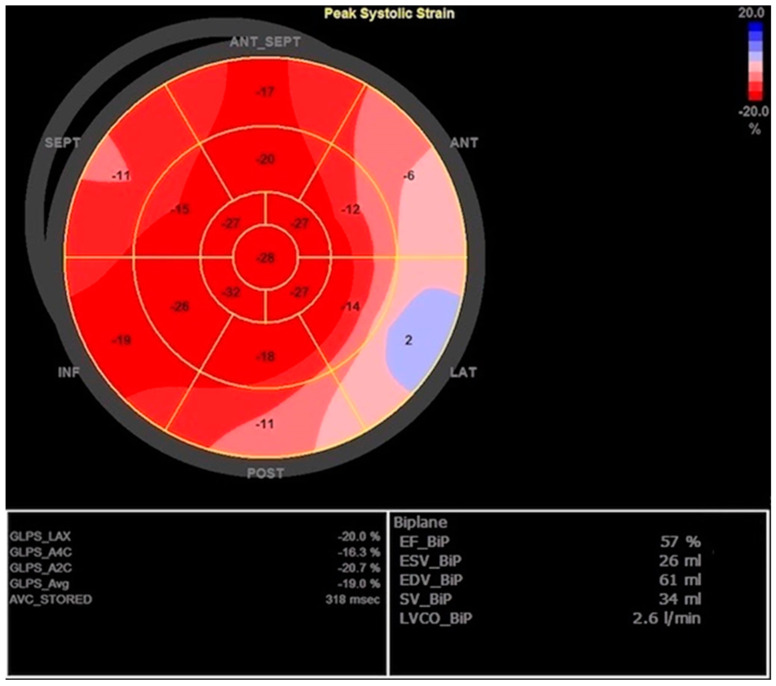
Second transthoracic echocardiography with improved left ventricle ejection fraction (EF) and improved global longitudinal peak strain (GLPS) but still noticeable reduced regional strain values.
